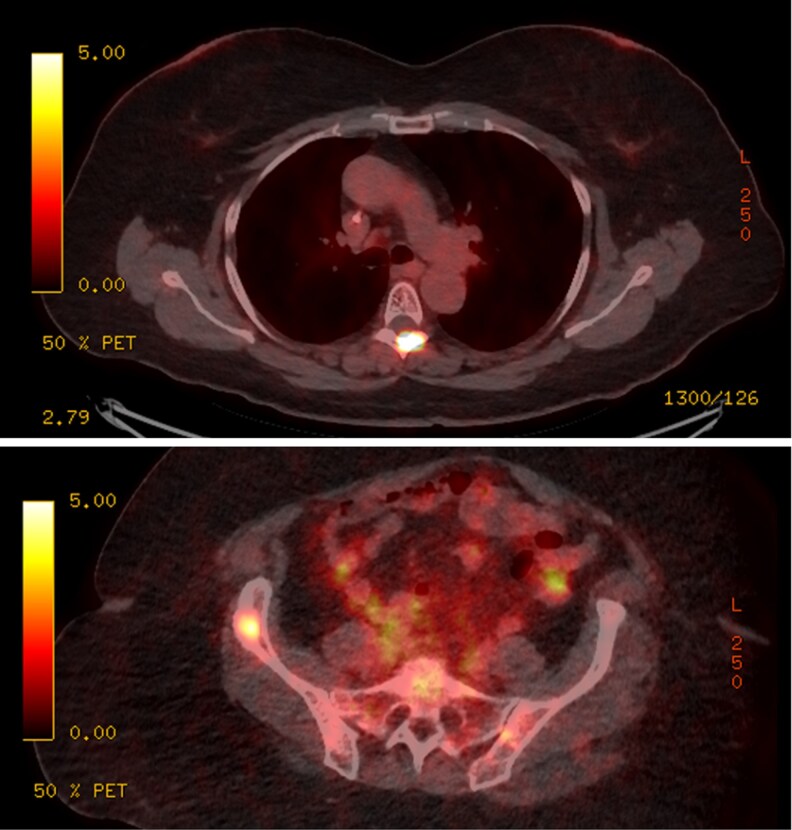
Case #3 ^68^Ga-DOTATATE PET/CT with intense radiotracer activity in (top) the left lamina of T5 vertebra and (bottom) anterior right iliac.
